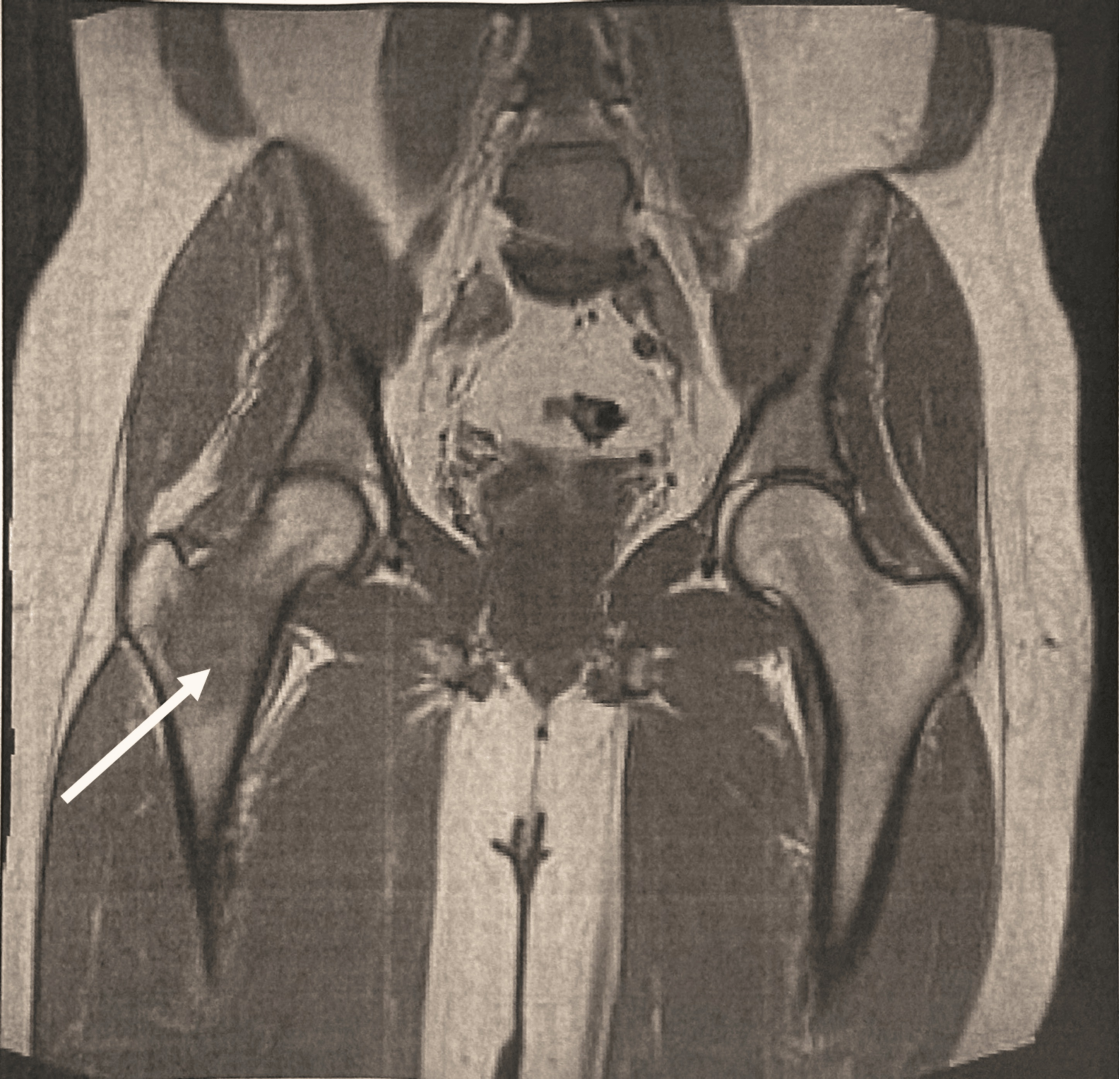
MRI of the Right Hip (Month 1) Infiltrative, intramedullary-based mass lesion within the right femoral neck.
